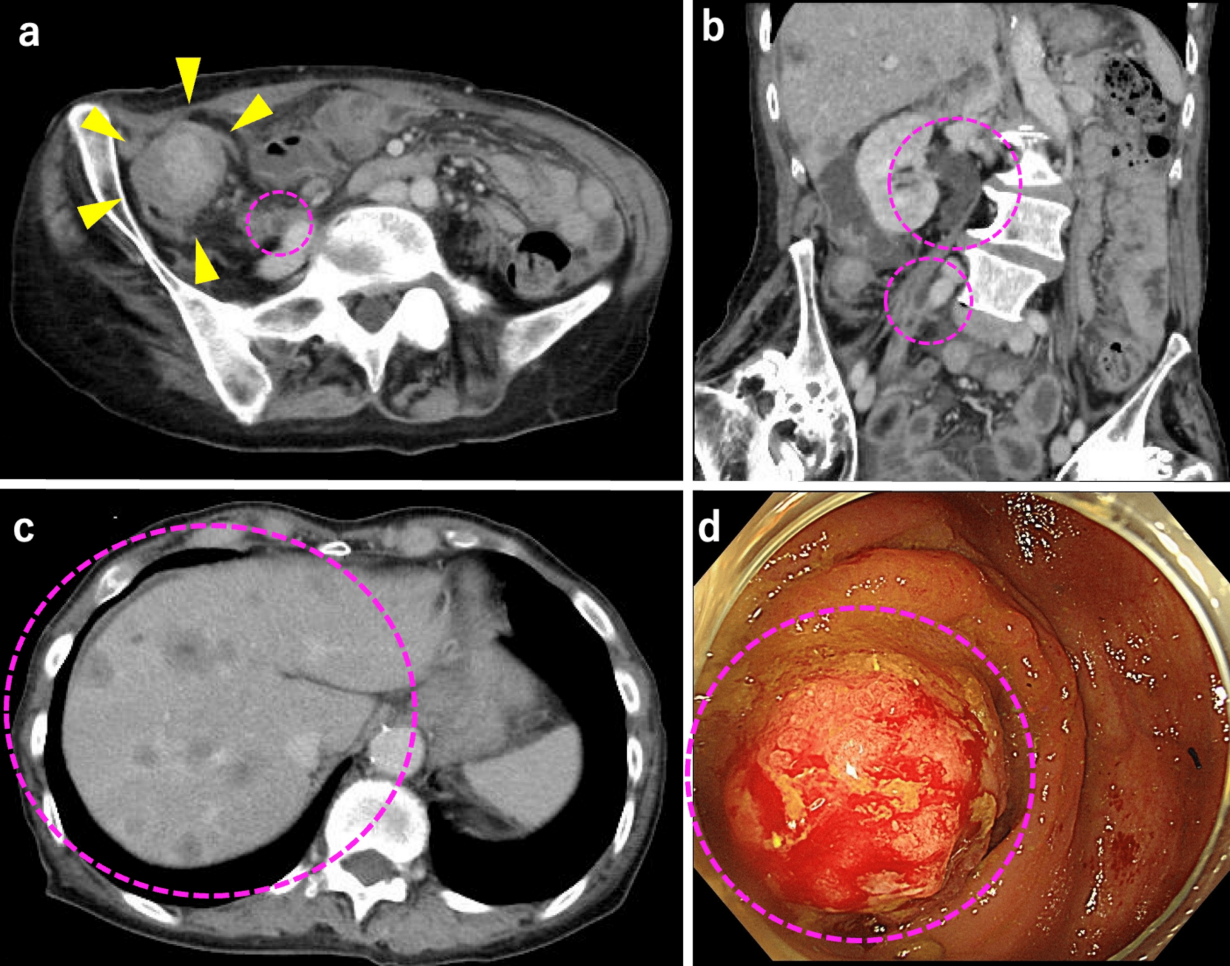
(a, b, c) computed tomography and (d) lower gastrointestinal endoscopy (a, b) Computed tomography (CT) revealed contrast-enhanced wall thickening extending from the cecum to the ascending colon. The right ureter showed contrast-enhanced wall thickening accompanied by hydronephrosis. (c) Multiple hypodense masses of varying sizes in the liver. (d) Lower gastrointestinal endoscopy showed a type 1 tumor in the ascending colon that obstructed the passage of the scope.
